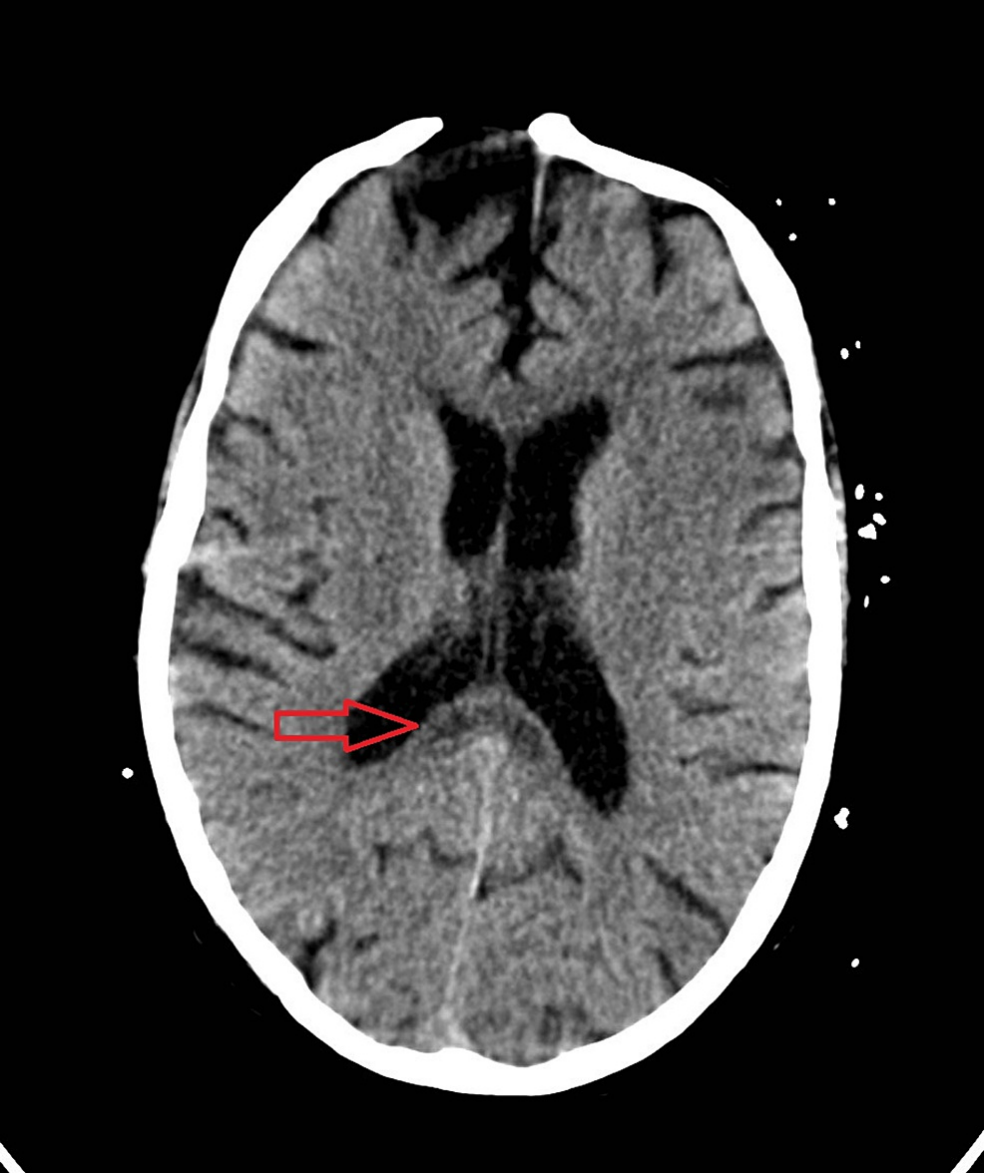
CT scan showing lesion in the splenium of the corpus callosum (arrow). Evidence of bone discontinuity and bilateral anterior frontal cortico-subcortical encephalomalacia. CT: computed tomography
